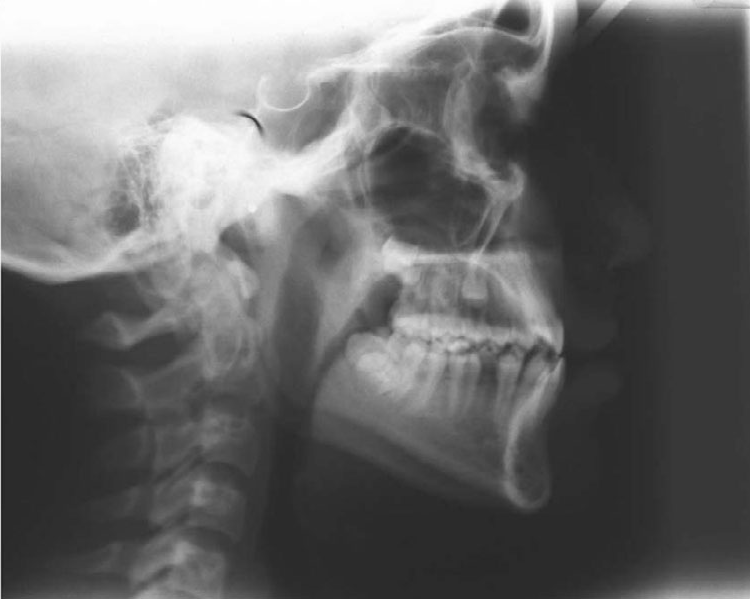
Pretreatment cephalometric radiograph of the patient.
